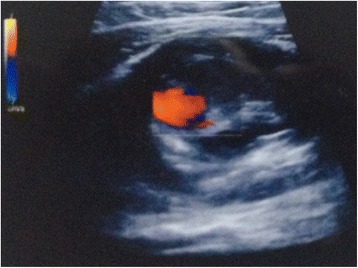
The color Doppler ultrasonography of the right lower limb showed the presence of the aneurysm at the right femoral superficial artery accompanied by peri-hematoma
